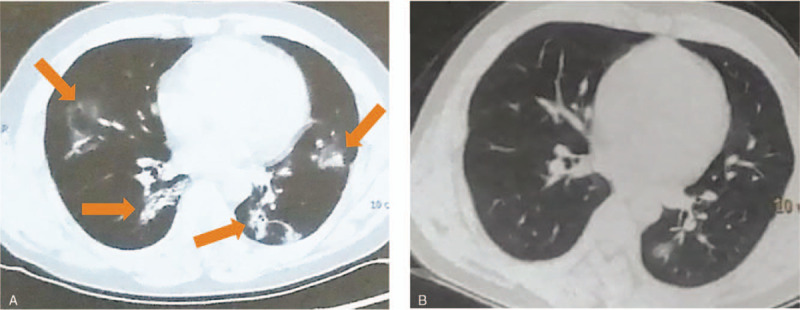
Chest CT images. (A) Exudative lesions were in the middle and lower lobe of the right lung and the lingular segment and lower lobe of the left lung. Middle lobe of the right lung and lingular segment of the left lung also showed ground glass opacity. Image was obtained on January 26th, 2020 (arrows). (B) After treatment, lesions in the middle and lower lobe of the right lung and lingular segment of the left lung were completely absorbed. Lesions in the lower lobe of the left lung were partially absorbed, manifested as a reduced lesion size, and density. Image was obtained on February 13th, 2020.
